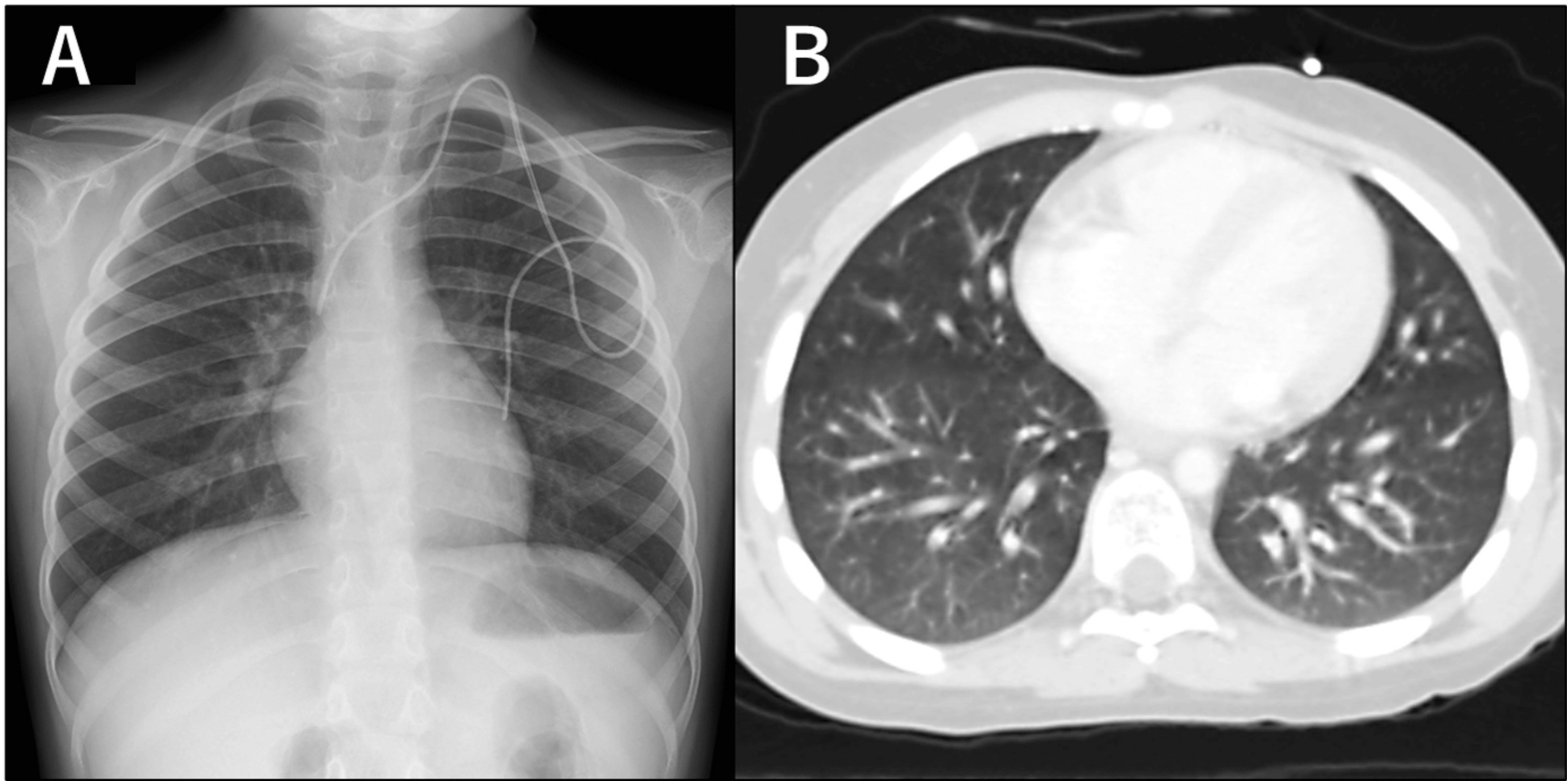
Radiologic improvement with minimal residual abnormalities after multimodal therapy (A) Chest radiograph obtained three months after initiation of therapy shows marked improvement of hyperinflation, with only minimal residual overinflation remaining. (B) Chest CT demonstrates near-complete resolution of previous air trapping, with predominantly normal lung fields CT: computed tomography
